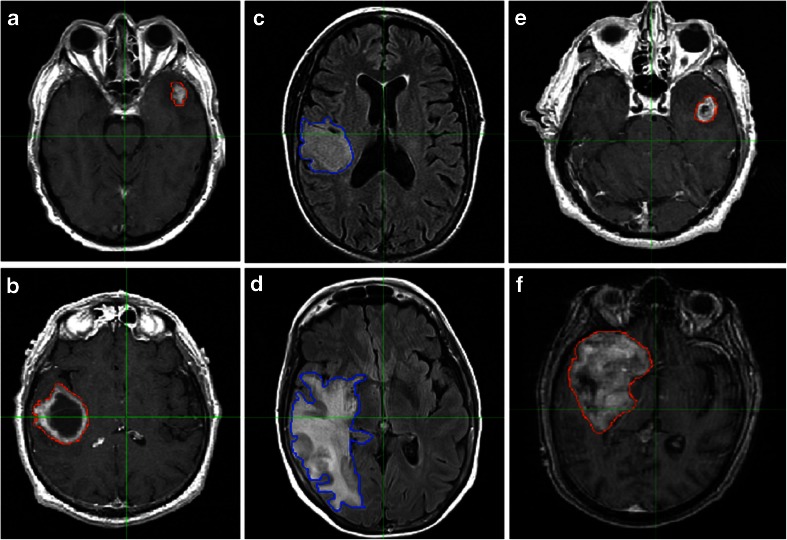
Examples of images characterized by various imaging features. Representative scans of low (a) and high (b) necrosis/total tumor volume ratios, low (c) and high (d) FLAIR/total tumor volume ratios, and low (e) and high (f) tumor bulk volumes are visualized. Masks outline areas used to determine various volumetric features used throughout the project (*red* for tumor bulk on T1-weighted post-Gd contrast (T1C) images, *blue* for T2-FLAIR hyperintensity on T2-weighted FLAIR images)
